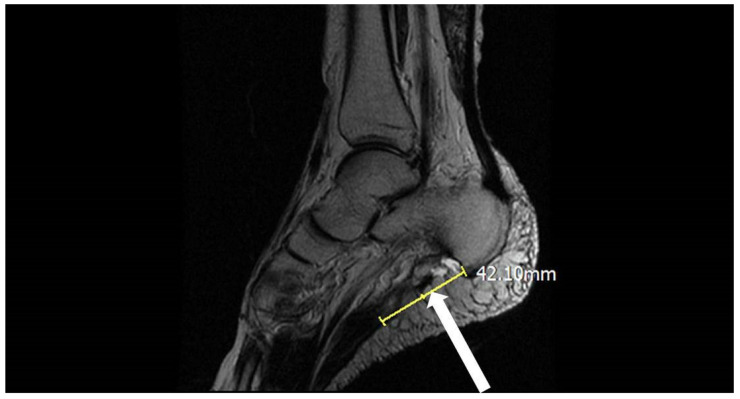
The white arrow indicates the complete rupture of the planta fascia with a 4.2-cm gap between the distal fascia stump and the calcaneal tuberosity on an MRI sagittal view.
